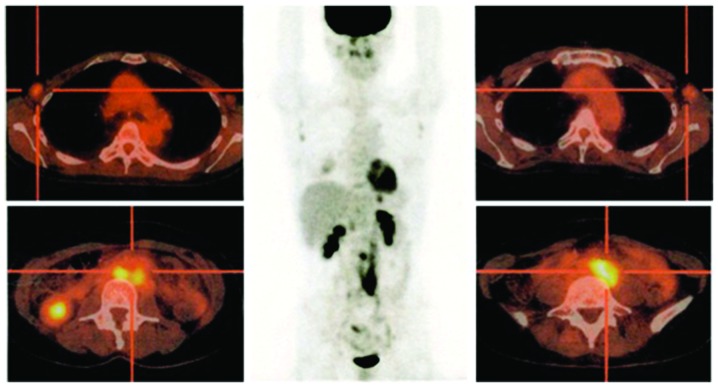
PET demonstrating an accumulation of ^18^F-fluorodeoxyglucose in the bilateral AxLNs (SUVmax, 2.8) and intra-abdominal para-aortic LNs (SUVmax, 5.8). PET, positron emission tomography; Ax, bilateral axillary; LN, lymph node; SUVmax, maximum standardized uptake value.
